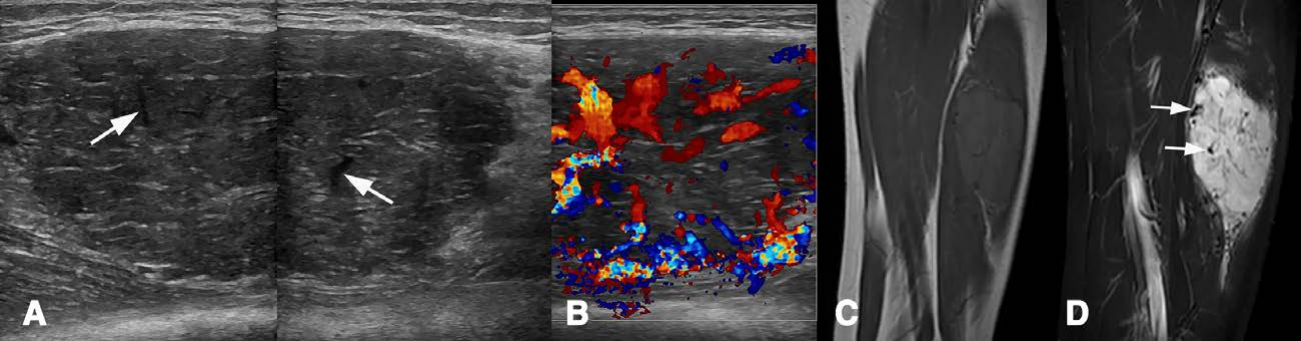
Sonography and MRI of the left thigh. (A) Gray scale ultrasound reveals a heterogeneous hypoechoic mass lesion in the vastus lateralis muscle, well limited, and with tube-like echoless in it (arrows). (B) Superb microvascular imaging shows abundant color flow signals in anechoic area in central and peripheral of the tumor. (C) Coronal T2-weighted fat-suppressed image shows high signal lesion in the vastus lateralis muscle, and multiple flow voids appeared both central and peripheral (arrows). MRI = magnetic resonance imaging.
